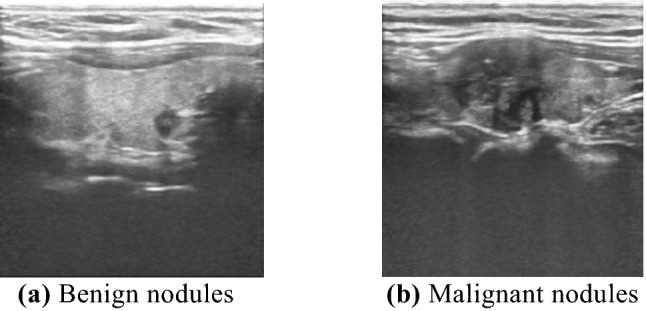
Ultrasonic images of nodules.
